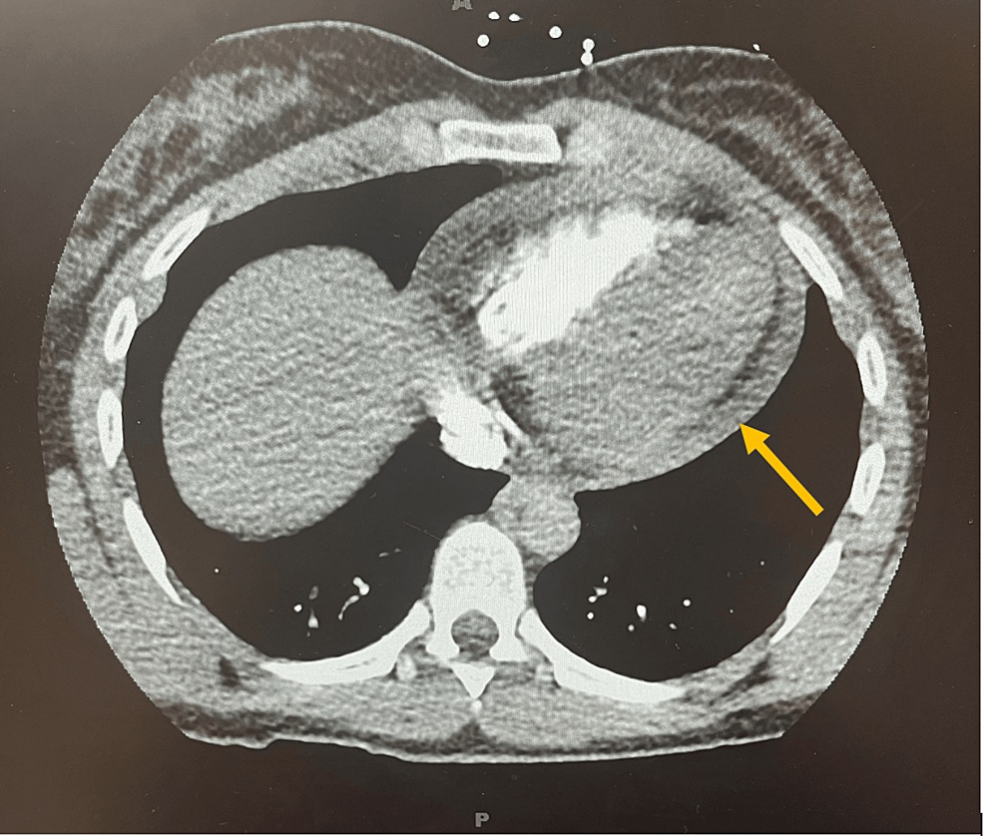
Chest computed tomography with angiography (CTA) showing a large pericardial effusion (yellow arrow).
